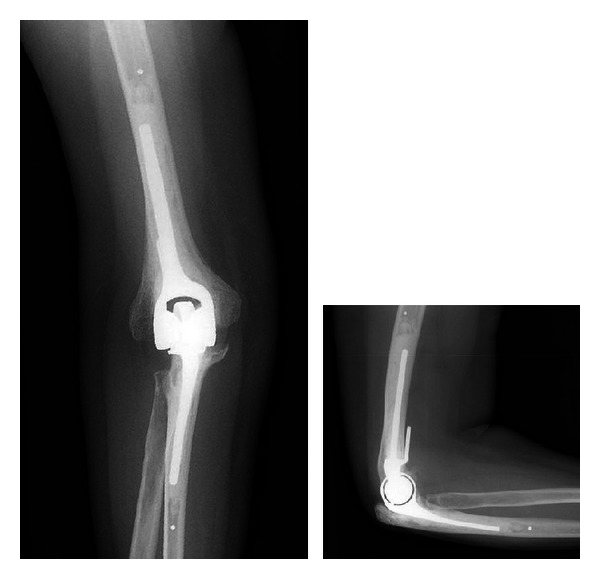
AP and lateral radiographs of a patient who underwent total elbow arthroplasty.
